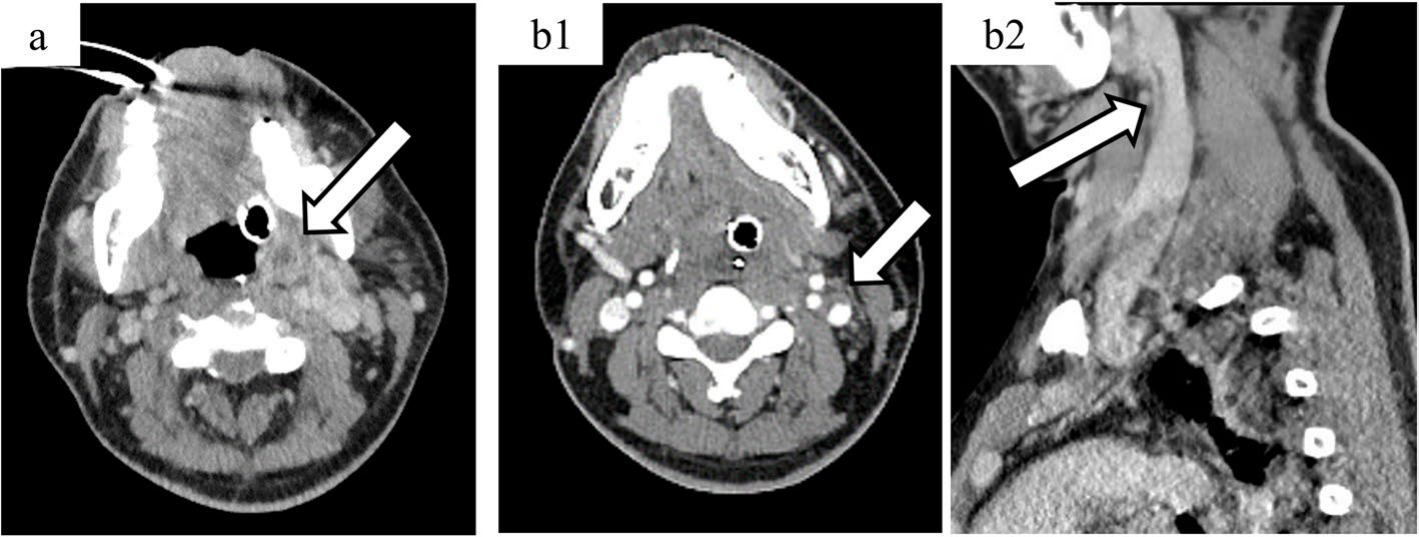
Neck contrast-enhanced computed tomography on day 10 of hospitalization. A peritonsillar abscess (4-a) and thrombus in the left internal jugular vein (4-b1 & 4-b2) were observed
